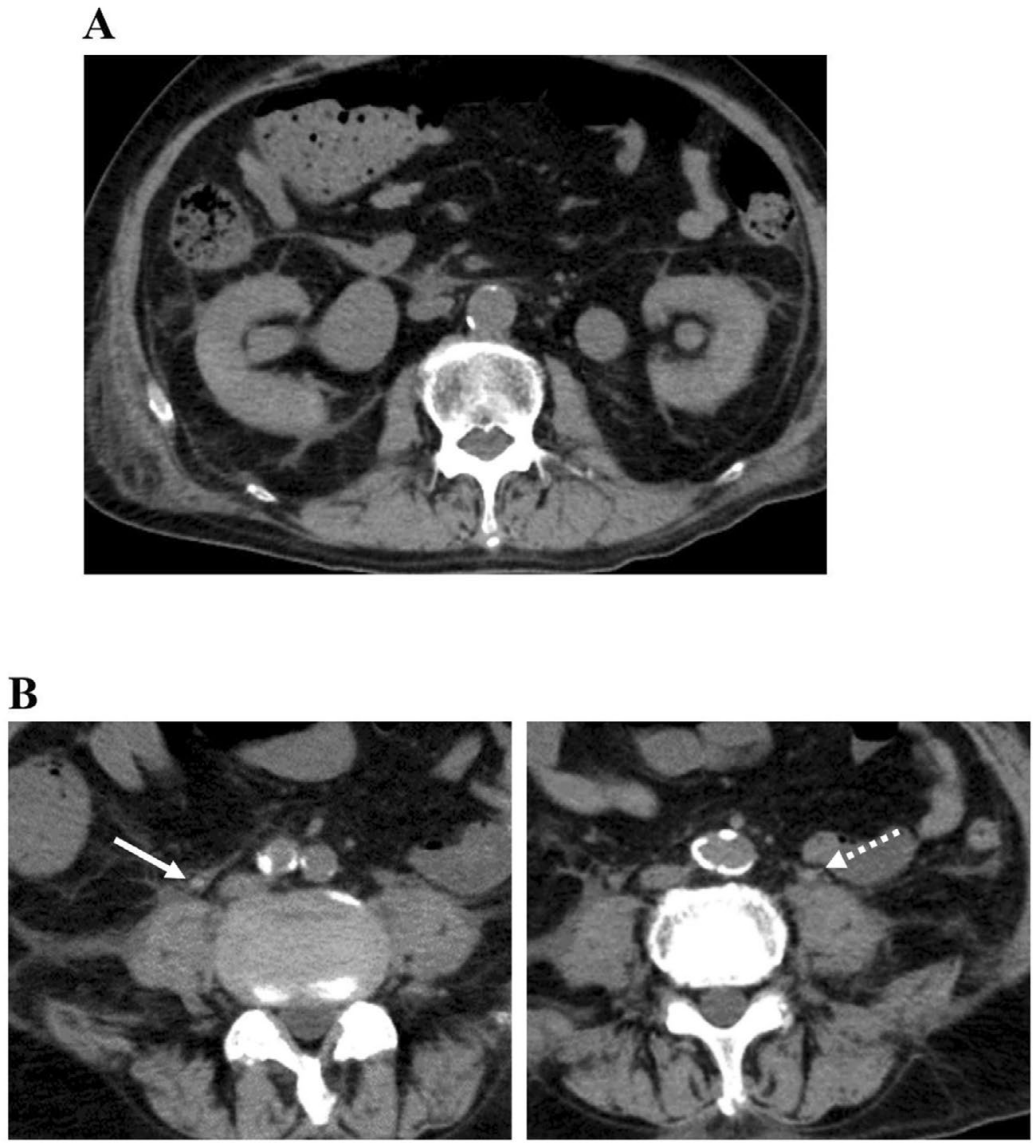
Abdominal non‐contrast CT scan images obtained for examination of worsened renal function. The causes of obstruction were (A) bilateral hydronephrosis and (B) presence of slightly dense masses in the examined sites (solid line arrow indicates right side, dotted arrow indicates left side).
